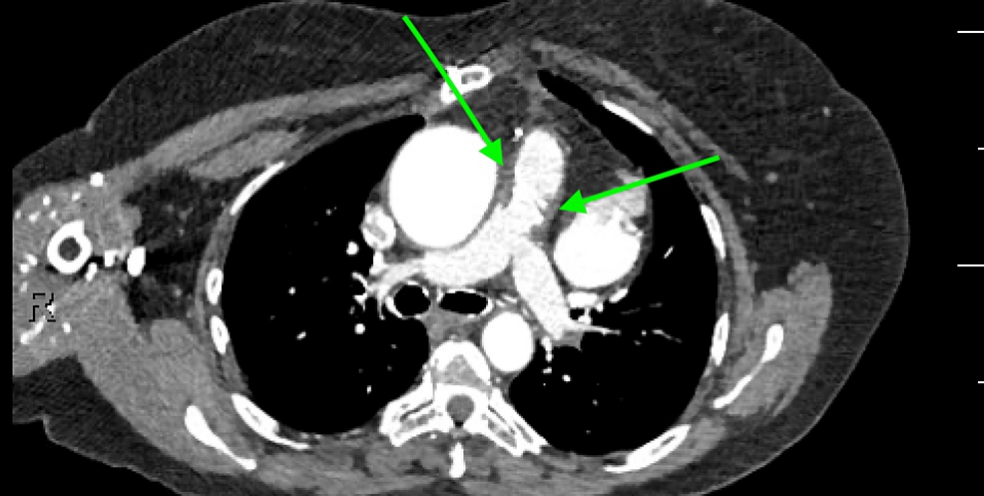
CT thorax with contrast. One-year postoperative evaluation showing stable pulmonary artery diameter and post-reconstruction changes compared to immediate postoperative evaluation Green arrows: post-reconstruction changes
